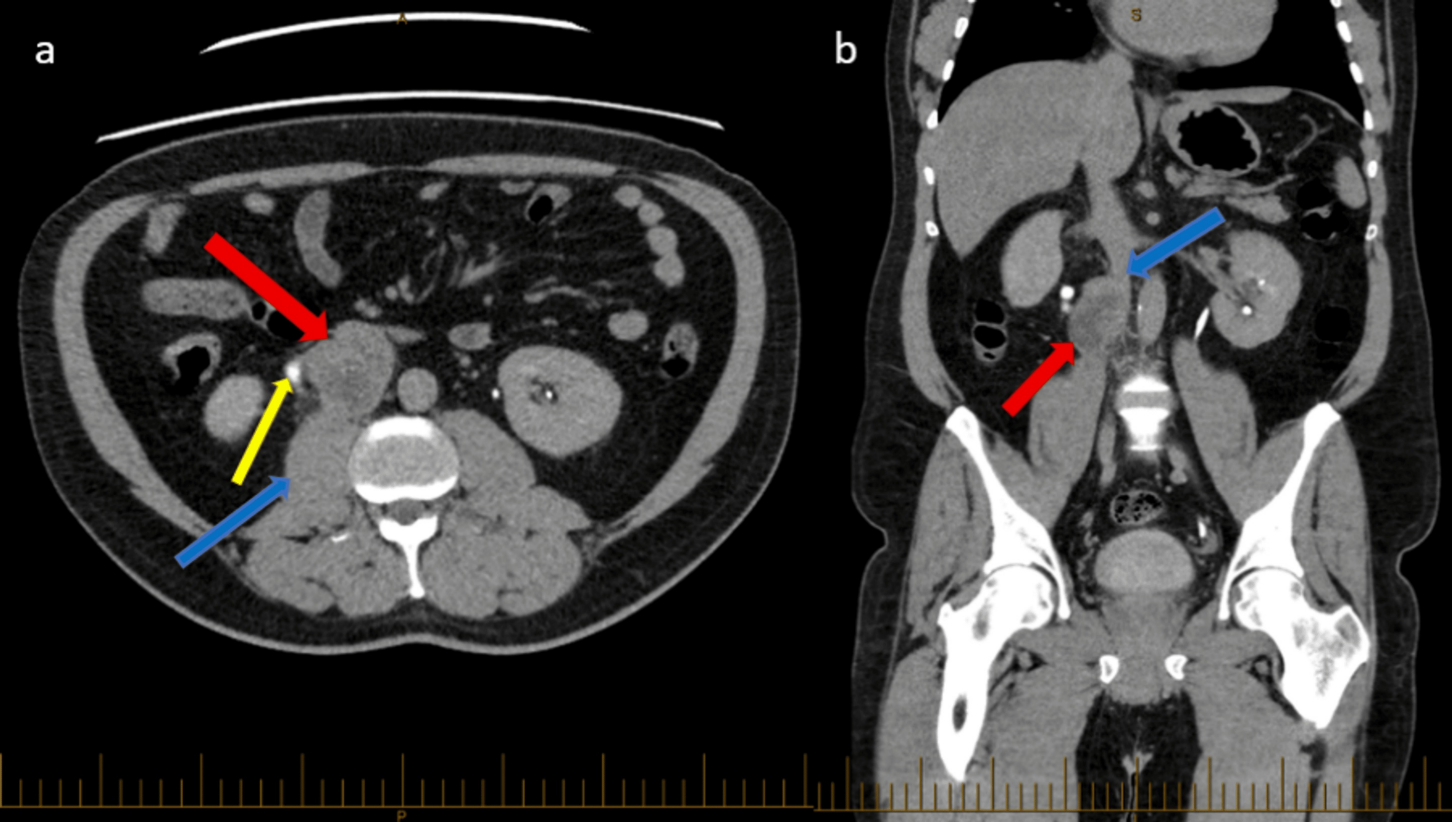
CT IVP delayed phase pre-operatively CT: computed tomography; IVP: intravenous pyelogram; IVC: inferior vena cava (a) Axial image demonstrating a 53 x 39 x 60 mm lobulated mass (red arrow) along the course of the right ureter (yellow arrow) with likely local invasion of the right anterior margin of the psoas muscle (blue arrow). (b) Coronal slice demonstrating the lobulated mass (red arrow) invading the IVC lumen (blue arrow)
